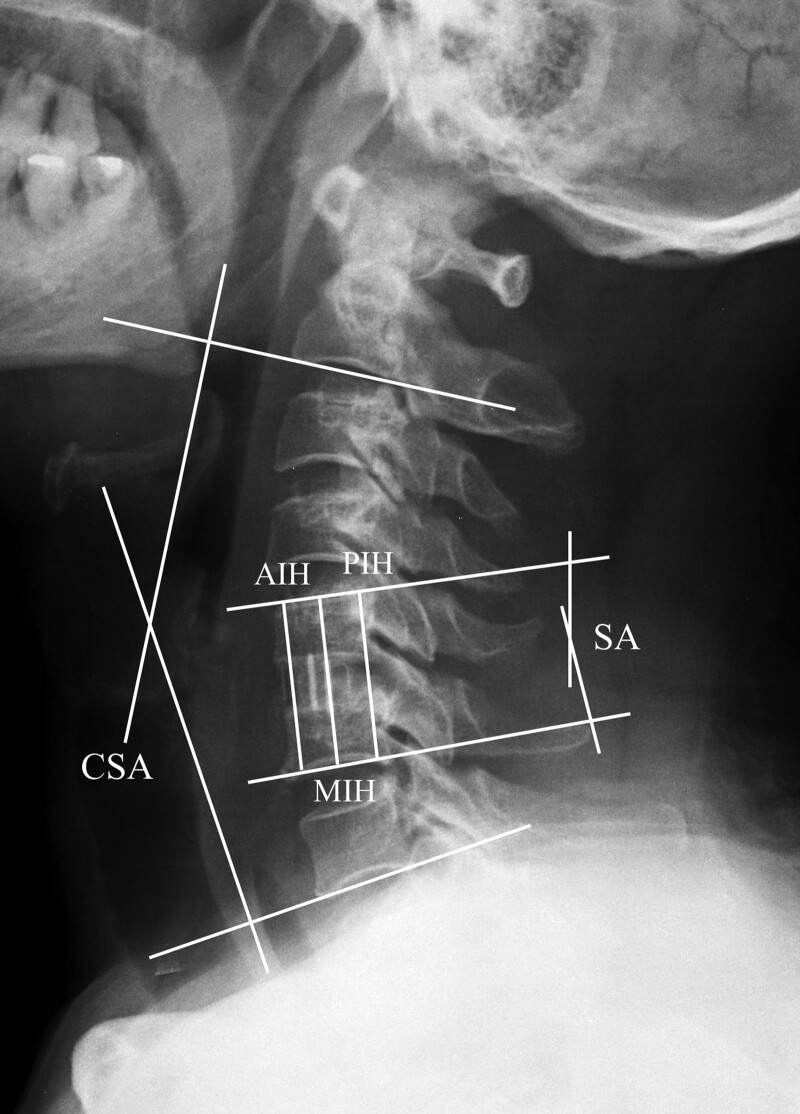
Cervical sagittal angle (CSA), segmental angle (SA), and anterior, middle, and posterior intervertebral height (AIH, MIH, and PIH) on lateral radiographs of the cervical spine.
